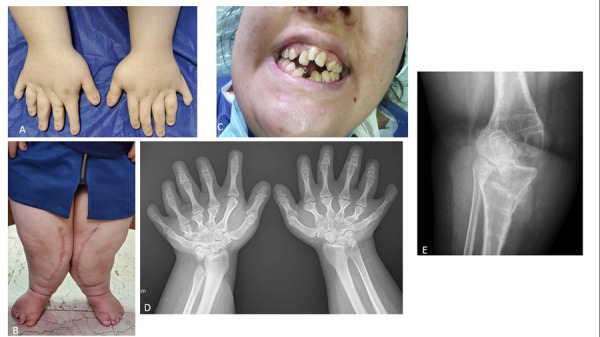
Clinical and radiologic manifestations of Ellis–van Creveld Syndrome. A: bilateral brachypolydactyly, hypotrophic fingernails. B: flat sole, pronated foot and hypotrophic nails. No polydactyly was seen in the toes. C: serrated incisal margins, dental transposition, diastema, conical teeth and enamel hypoplasia. D: radiographic features of upper extremity including postaxial polydactyly and polymetacarpia, enlarged distal radius and fusion of capitate and hamate. E: Knee valgus is evident despite previous surgery.
